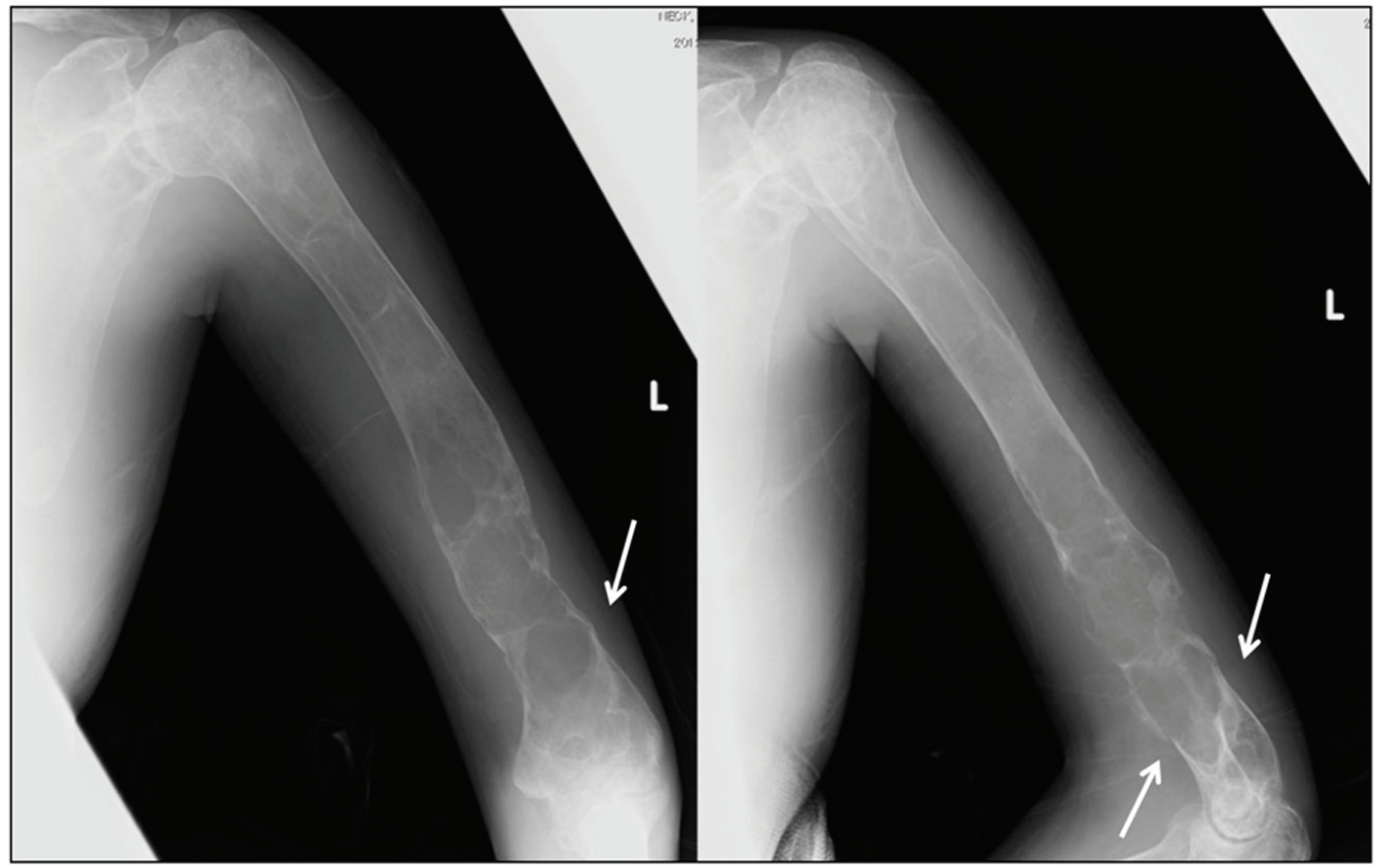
X-ray images of the left humerus at the presentation. Plain radiography shows osteolytic lesions with slightly sclerotic septa in the left humeral shaft and metaphysis. A supracondylar fracture (arrow) is evident.
